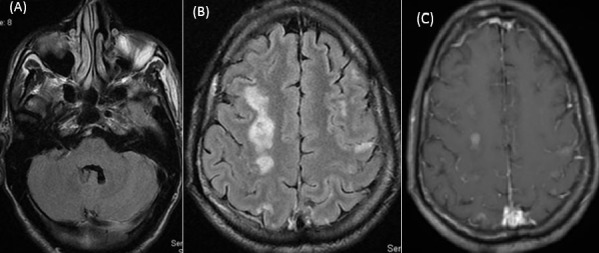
(A,B) at follow-up examination, Two week after the onset of illness. In comparison with the first examination, T2-FLAIR images show resolution of cerebellum hyperintensities,and minimal decrease in the size of the lesion of occipital, frontal and right semiovale centrum white matter; (C) the unenhanced microhaemorrhagic lesionson contrast-enhanced T1-weighted images
